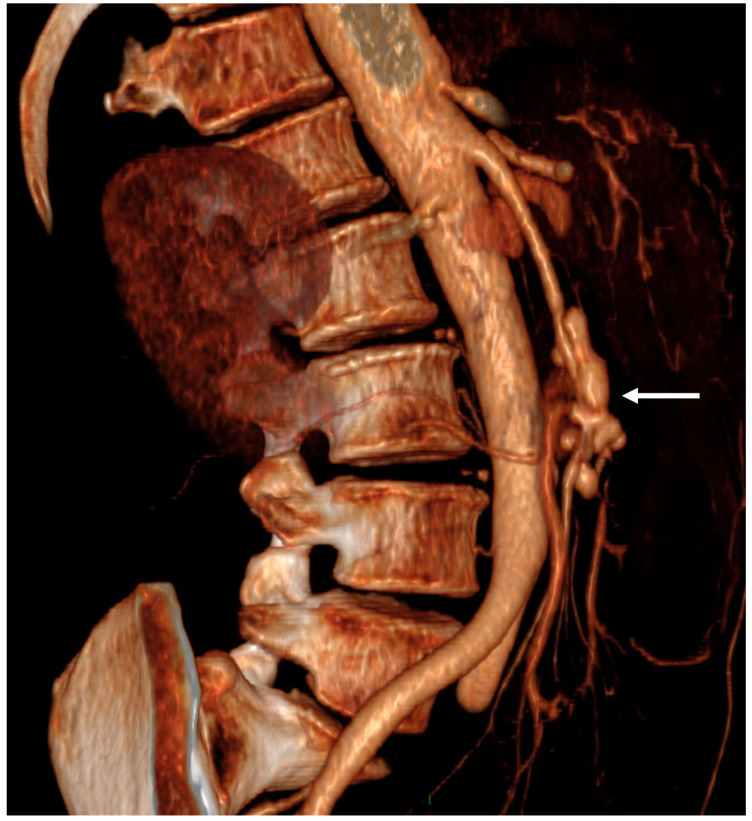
Superior mesenteric infectious aneurysm in a *Streptococcus gordonii* infectious endocarditis. A 55-year-old woman was admitted for a two-month history of fever, fatigue, arthralgia and worsening abdominal pain for one week. Her medical history included hypertension. Abdominal examination at admission showed non-tender abdomen. Transthoracic and transesophageal echocardiography (TTE/TEE) revealed 2 mitral valve vegetations (14 and 25 mm) and severe regurgitation. Blood cultures grew *Streptococcus gordonii*. Antimicrobial therapy with amoxicillin and levofloxacin was initiated. Abdominal CT-angiography (CTA) showed a great lobulated aneurysm (55 mm in maximum diameter) of the superior mesenteric artery (volume rendering, VR, arrow).
